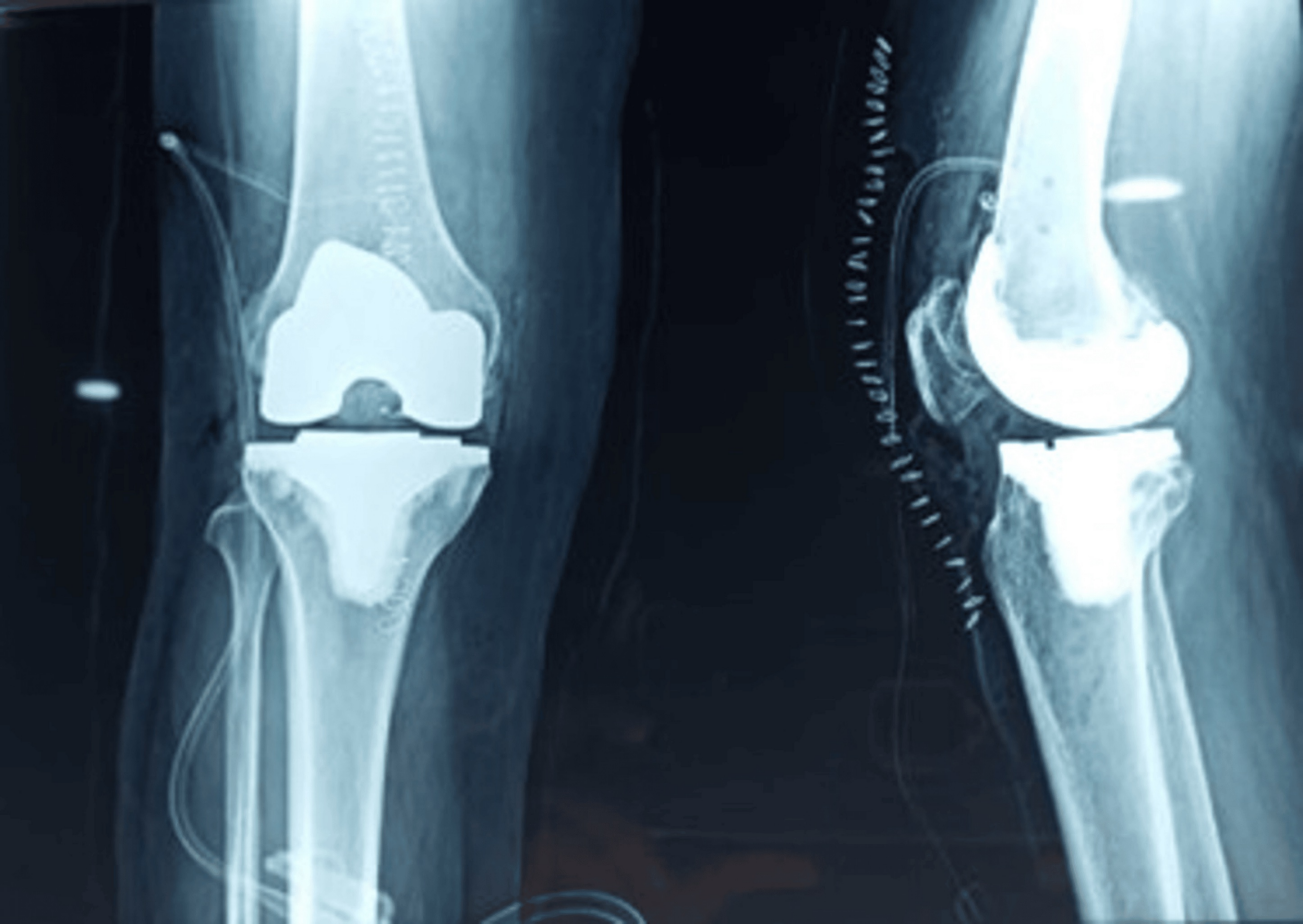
Post operative X-ray. Image Credit (Corresponding author): Dr. Sanjay B. Londhe
